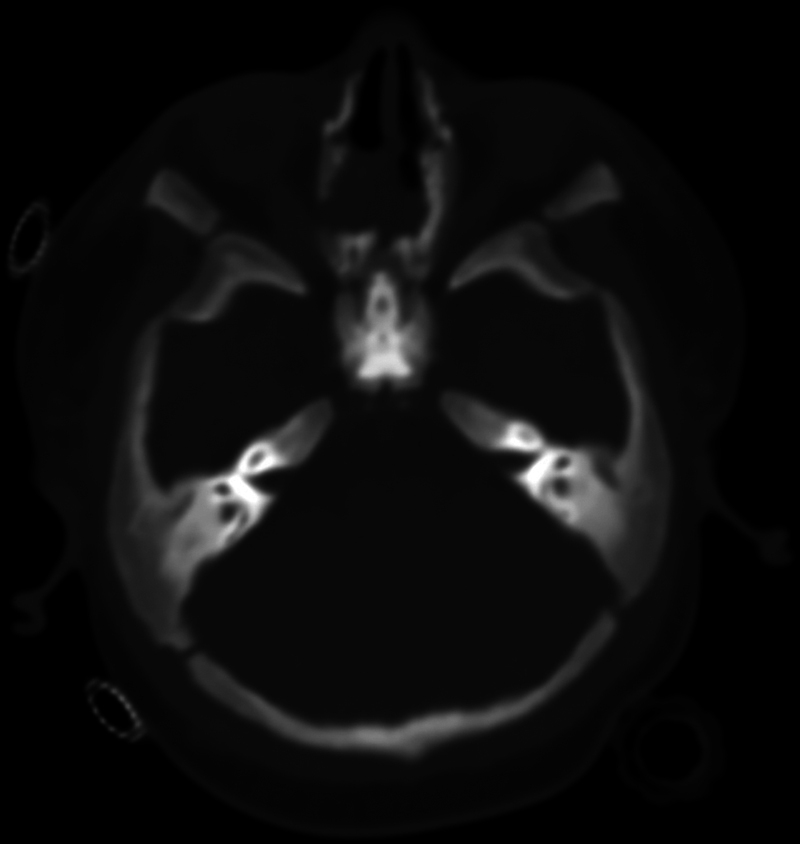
Computed tomography brain showing decompressed optic canal on the left on postoperative day 9.
